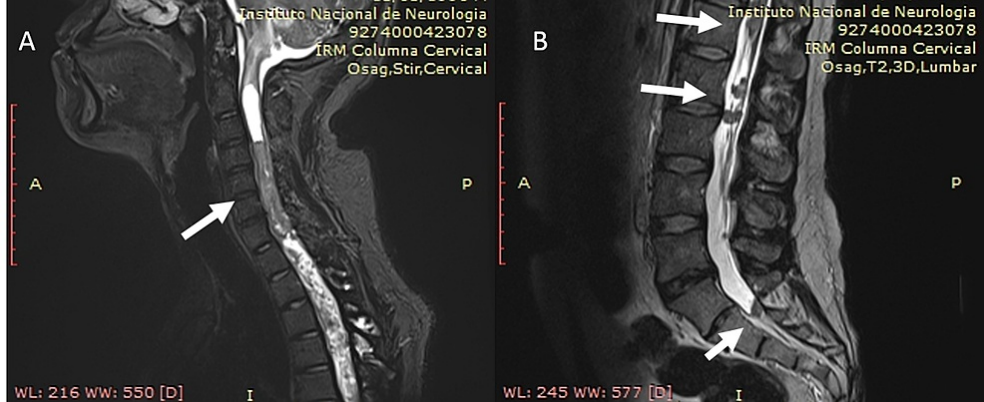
A) cranial and B) lumbar MRI showing multiple implants.
